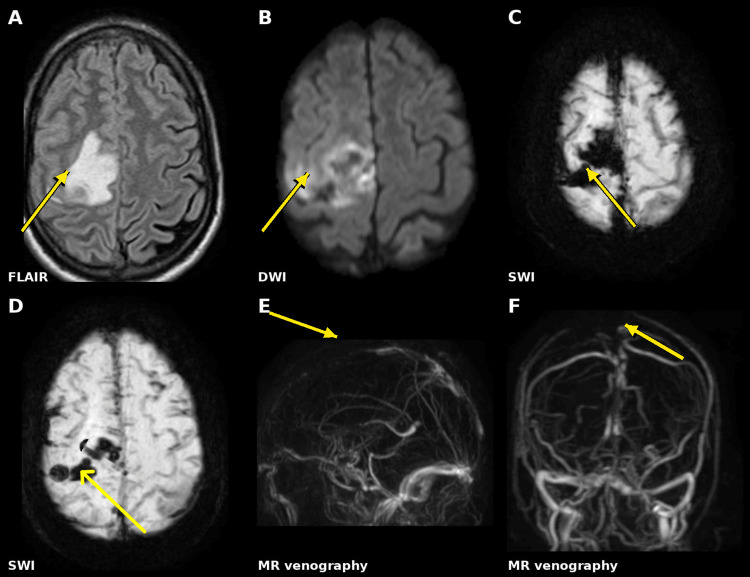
Multimodal magnetic resonance imaging demonstrating scrub typhus-associated superior sagittal sinus thrombosis with hemorrhagic venous infarction. Axial FLAIR (A, yellow arrow) shows a hyperintense lesion in the right parasagittal fronto-parietal region consistent with a venous infarct. Diffusion-weighted imaging (B, arrow) demonstrates corresponding hyperintensity, confirming acute injury. Susceptibility-weighted imaging at two contiguous axial levels (C and D, arrows) shows extensive blooming with discrete, rounded hypointense foci within the venous infarct, representing intralesional hemorrhage. Lateral (E, arrow) and coronal (F, arrow) MR venography demonstrate non-visualization of the superior sagittal sinus with prominent collateral cortical veins, confirming sinus thrombosis. FLAIR, fluid-attenuated inversion recovery; DWI, diffusion-weighted imaging; SWI, susceptibility-weighted imaging; MR, magnetic resonance; SSS, superior sagittal sinus.
